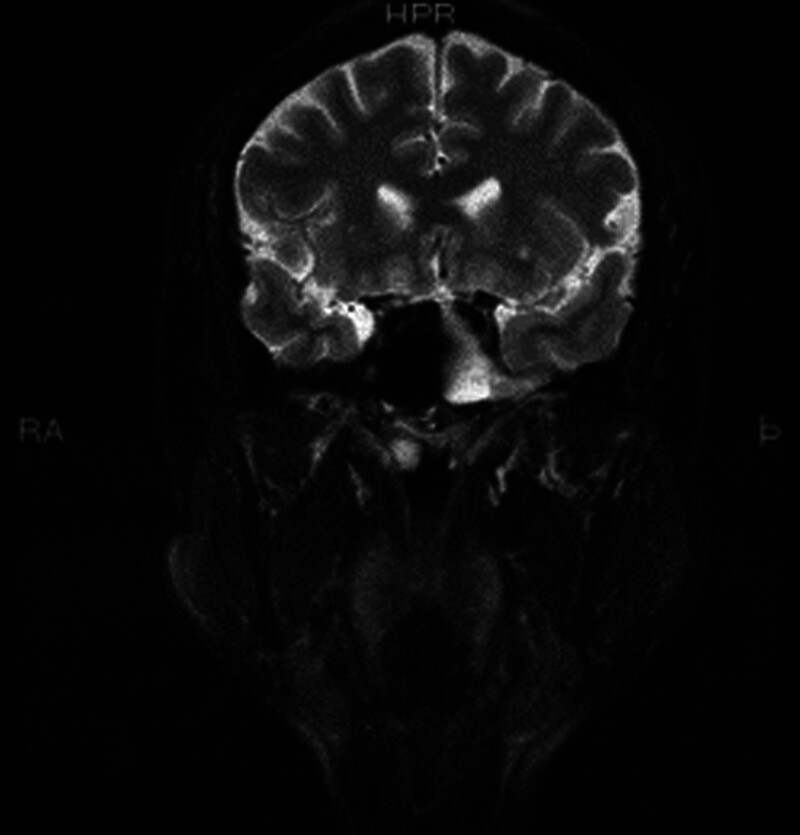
A 49-year old male patient with preoperative coronal map of sinus MRI. MRI = magnetic resonance imaging.
